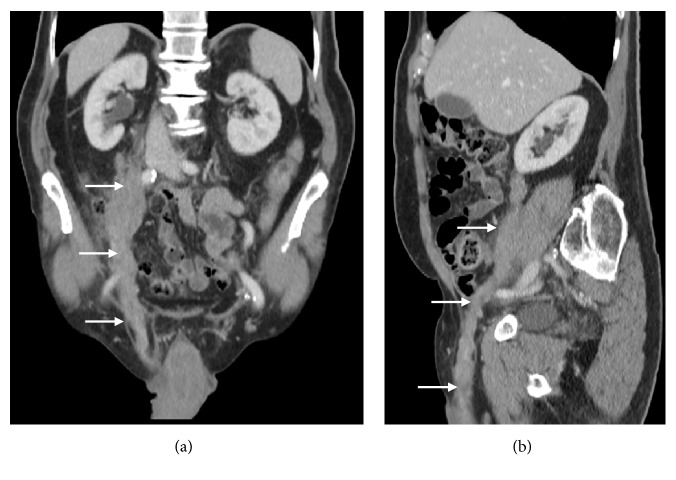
(a and b) Coronal and sagittal reformatted CT images of the abdomen and pelvis with contrast which demonstrate cord-like soft tissue extension from the right testicle into the retroperitoneum along the spermatic cord and gonadal vein.
